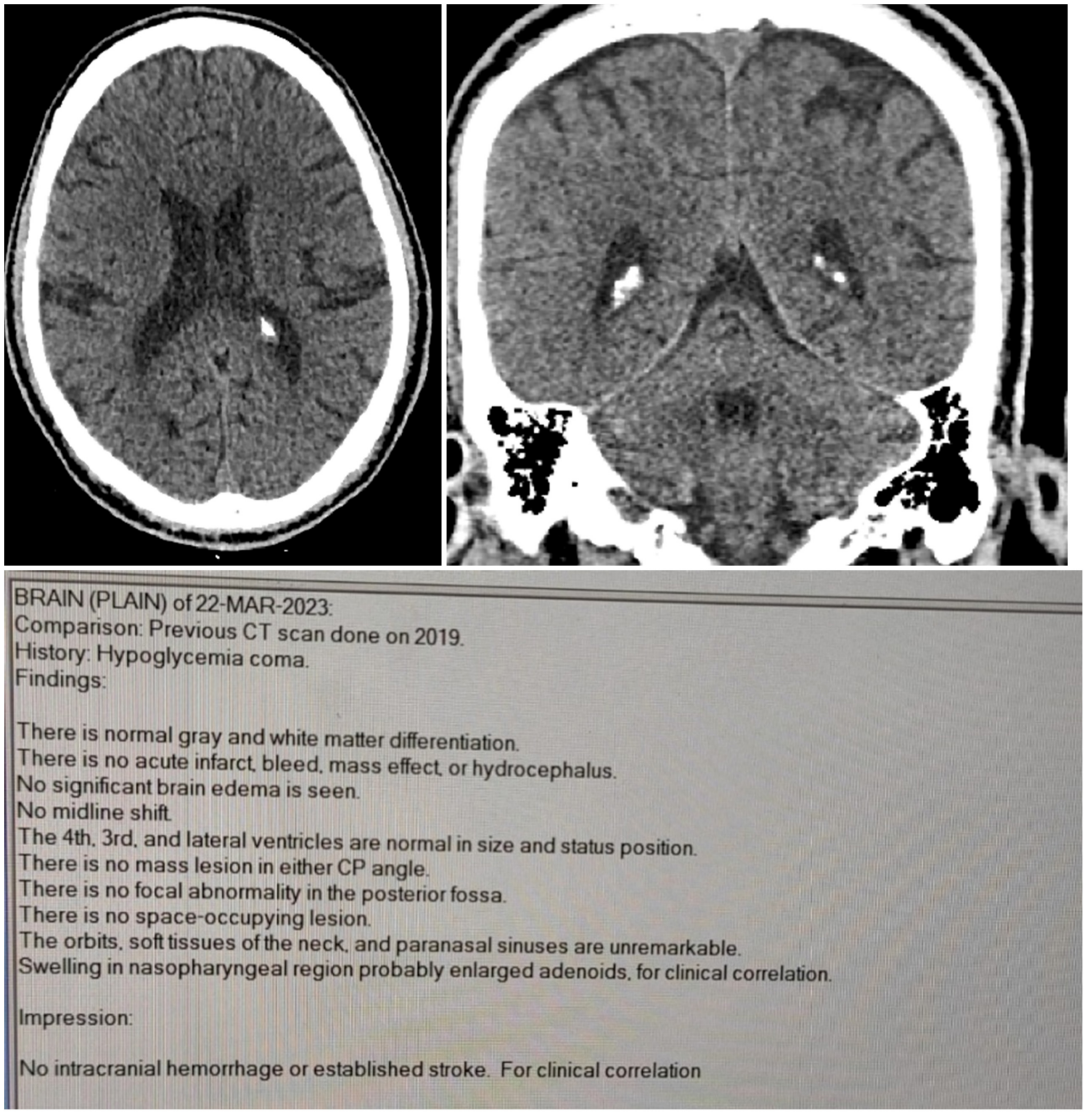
Non-contrast head CT. No acute intracranial hemorrhage, mass effect, or midline shift; ventricles normal in size and configuration.
